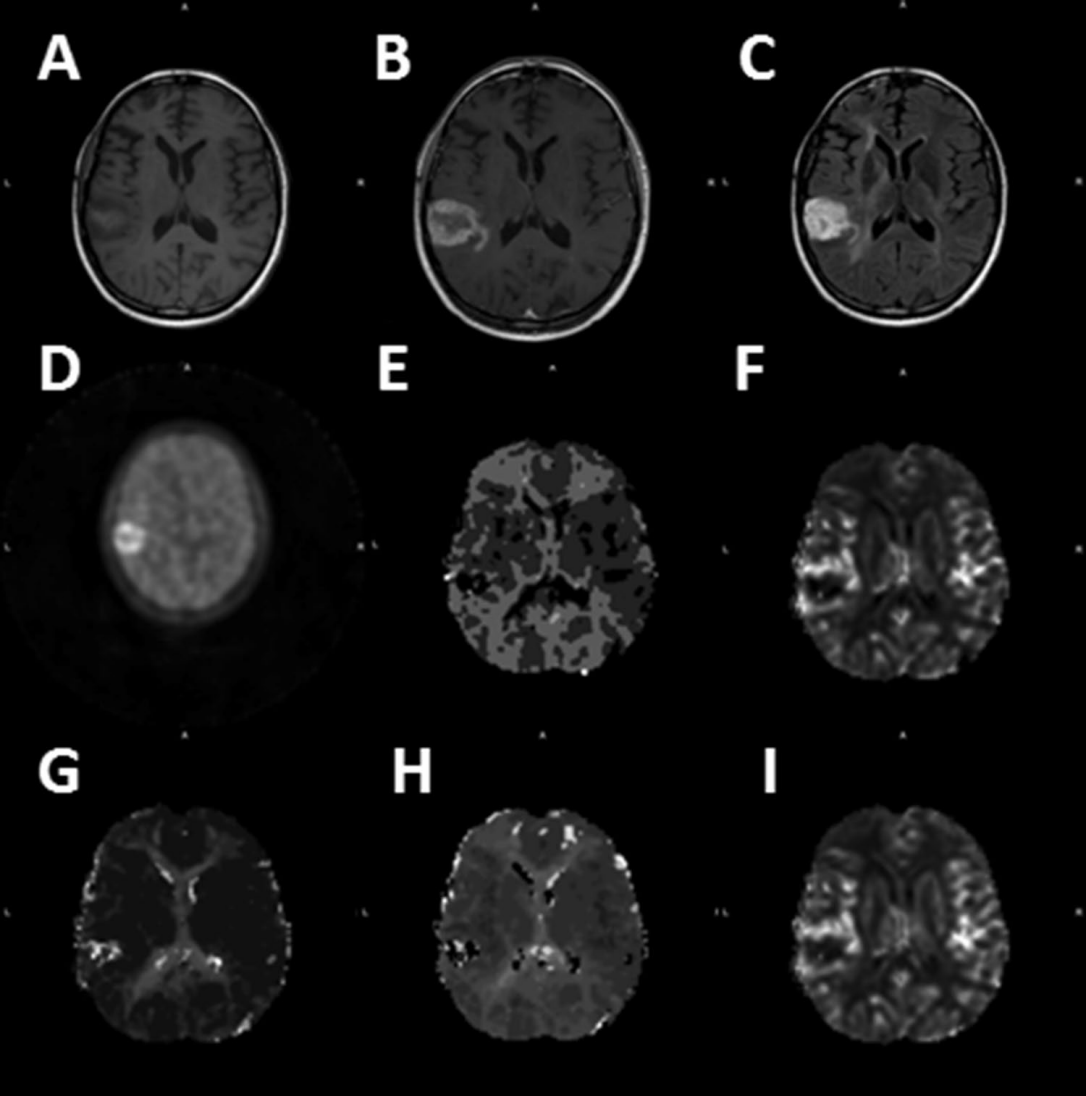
Representative images at baseline (Case UT003). (A) pre-contrast T1, (B) post-contrast T1, (C) FLAIR, (D)
